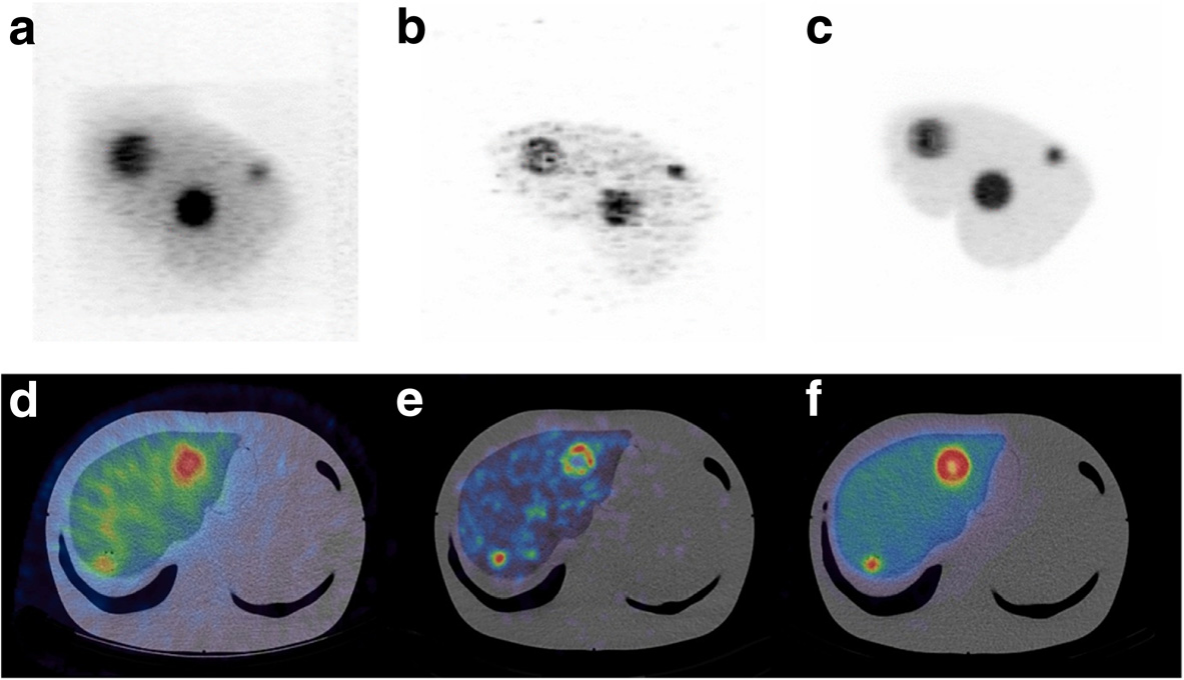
Y-90 SPECT/CT, MIP image (a) and fused transaxial slices (d). Y-90 PET/CT, MIP image (b) and fused transaxial slice (e). Tc-99m SPECT/CT, MIP image (c) and fused transaxial slice (f). Transaxial slices correspond to a plane intersecting the 20- and the 40-mm shell inserts
